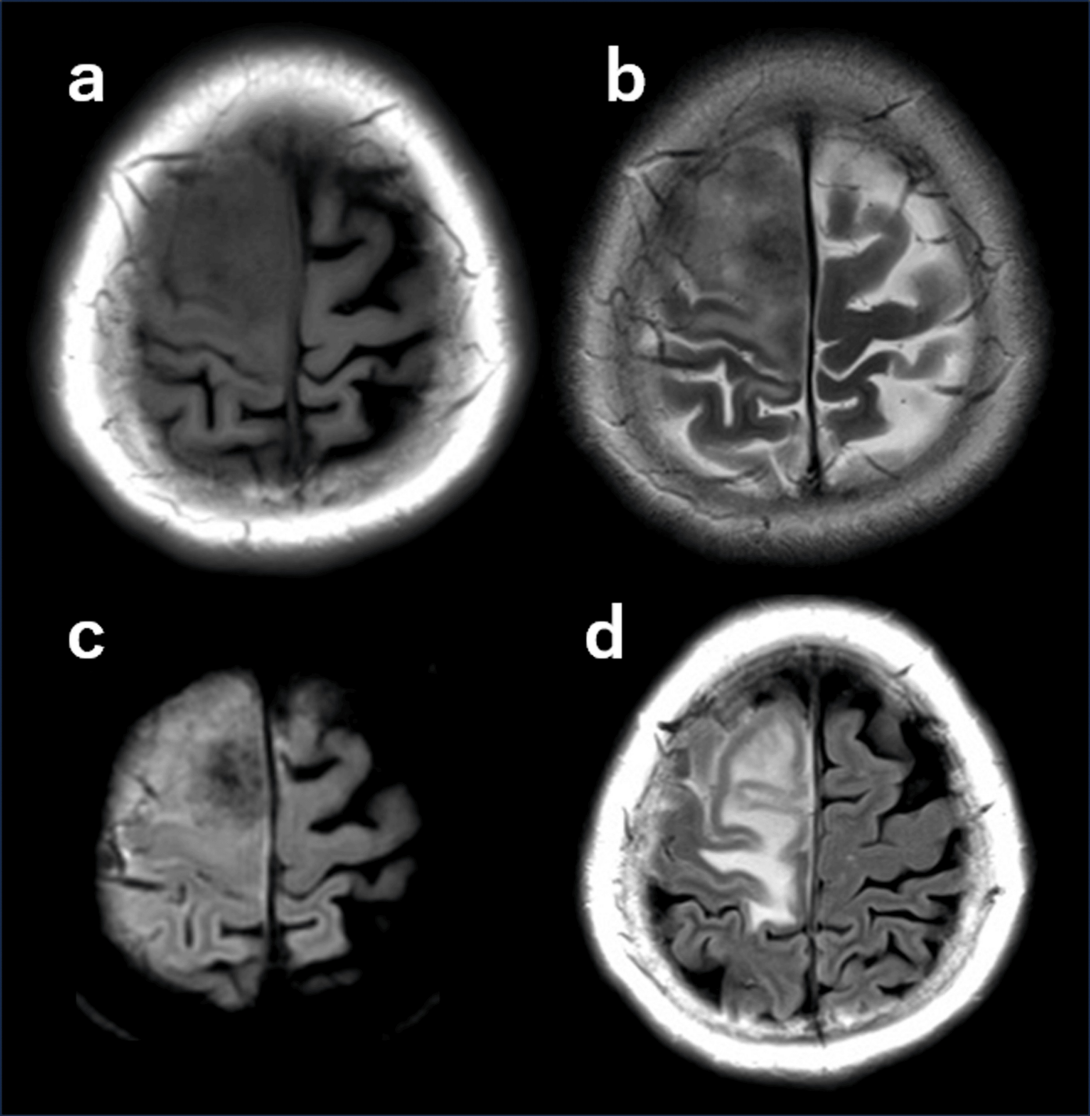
Preoperative magnetic resonance imaging. (a) T1-weighted image. (b) T2-weighted image. (c) Diffusion-weighted image. (d) Fluid-attenuated inversion recovery.
